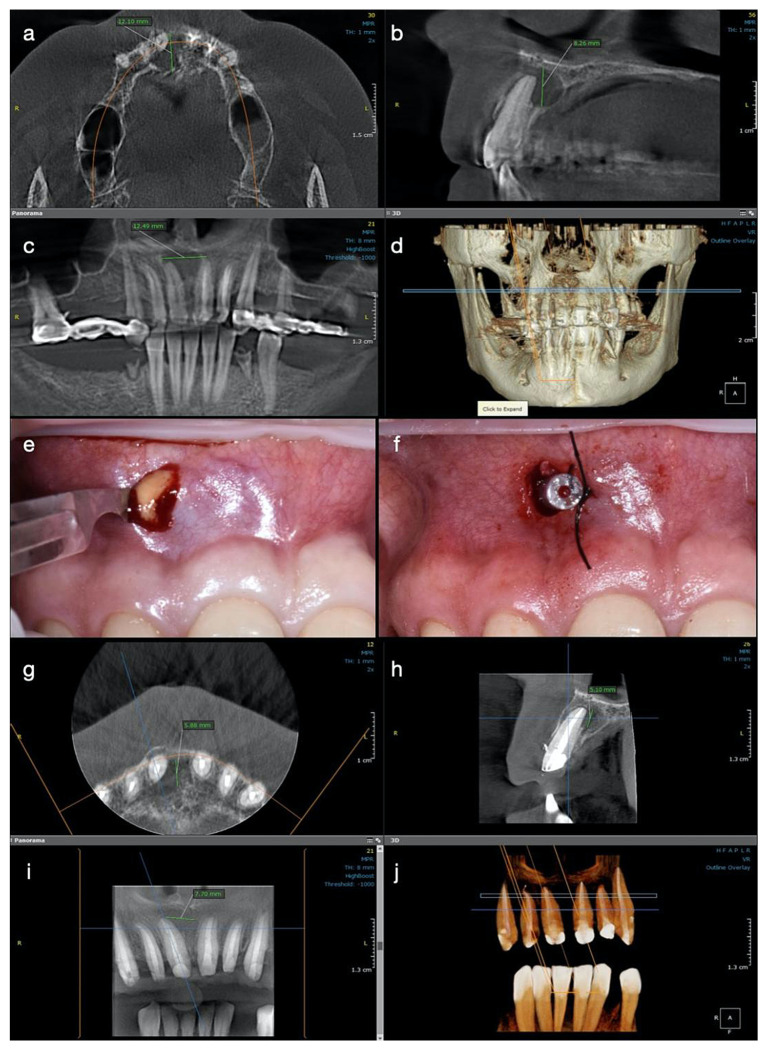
Case 2. Radiological investigations and clinical images of tooth #11: (a–d) Initial CBCT scans with the measurements of the lesion size: 12.10 mm in buccolingual direction, 8.26 mm in height, and 12.49 mm mesiodistal diameter, with the buccal cortical plate resorbed (12.10 × 8.26 × 12.49 mm); (e,f) The surgical procedure of decompression at the end of the endodontic treatment with the appearance of yellow fluid draining from the lesion, and the drainage tube fixed with sutures on the mucosa; (g–j) CBCT scans and the 3D reconstruction at 6 months after the endodontic therapy, with the formation of new bone and increased reduction of the lesion size, starting from its periphery (5.88 × 5.10 × 7.70 mm).
